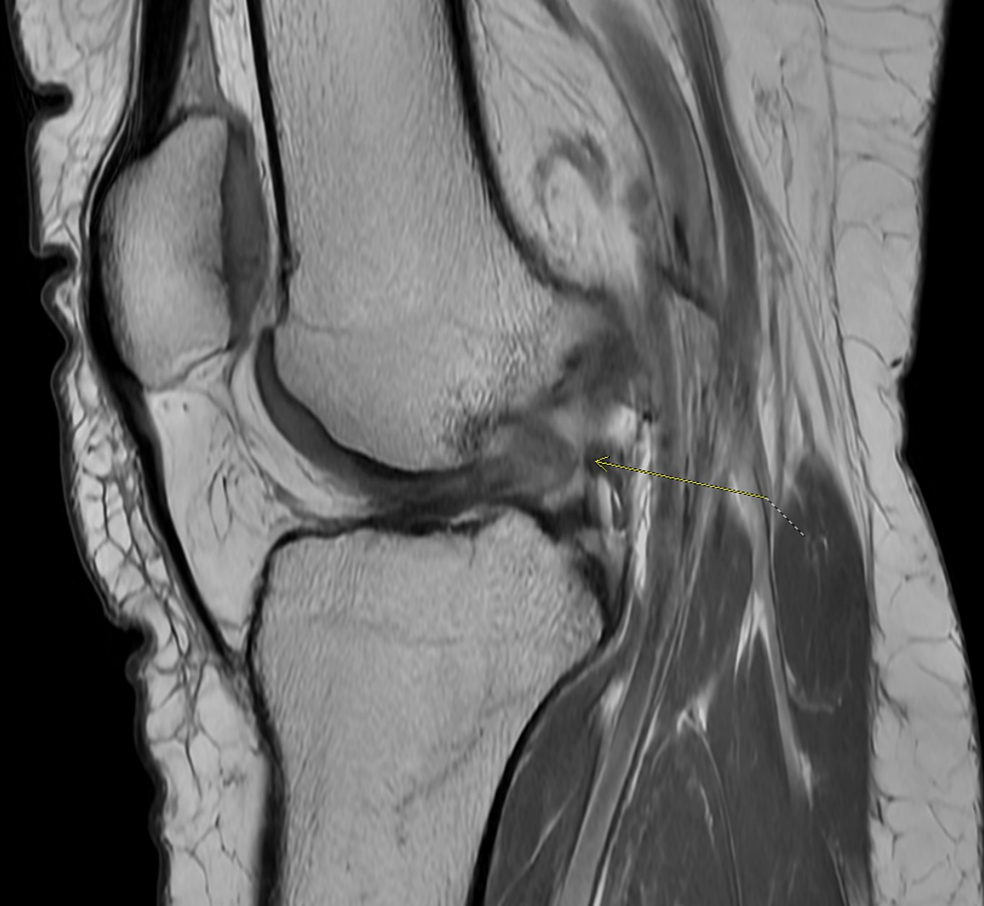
Sagittal MRI of the patient's right knee demonstrating ACL rupture. ACL: anterior cruciate ligament
